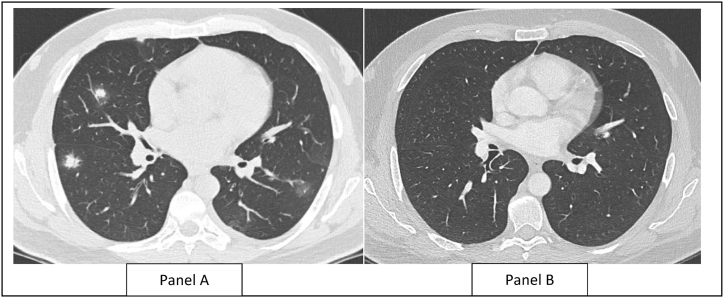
Computed Tomography Chest Images of Pulmonary window at the level of outset of segmental bronchus. Panel A: Variable sized nodular and patchy part solid opacities with surrounding ground glass opacification, showing subpleural and peribronchovascular predominance distribution. Panel B: Resolution of the opacities.
